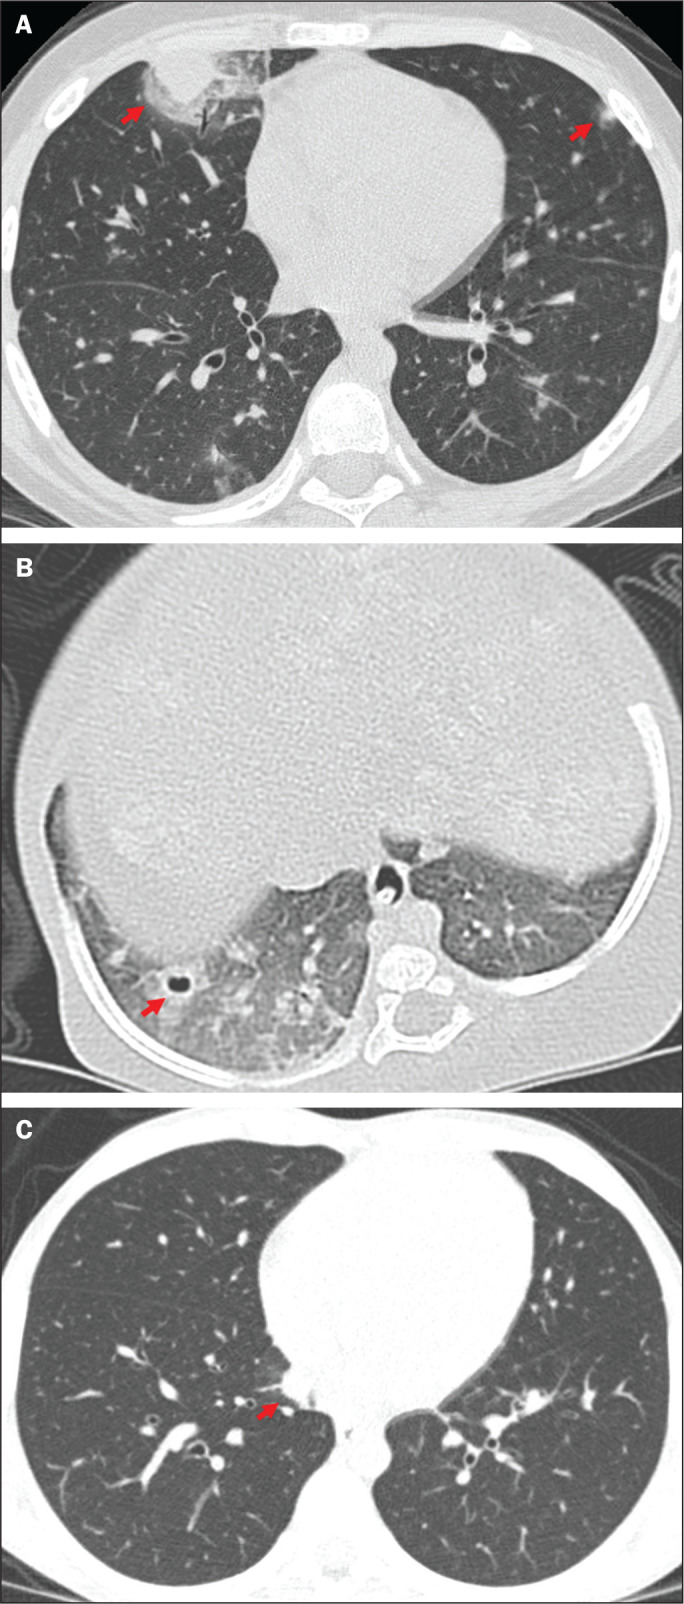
Findings typical of IFI on chest CT scans: multiple nodules with the halo
